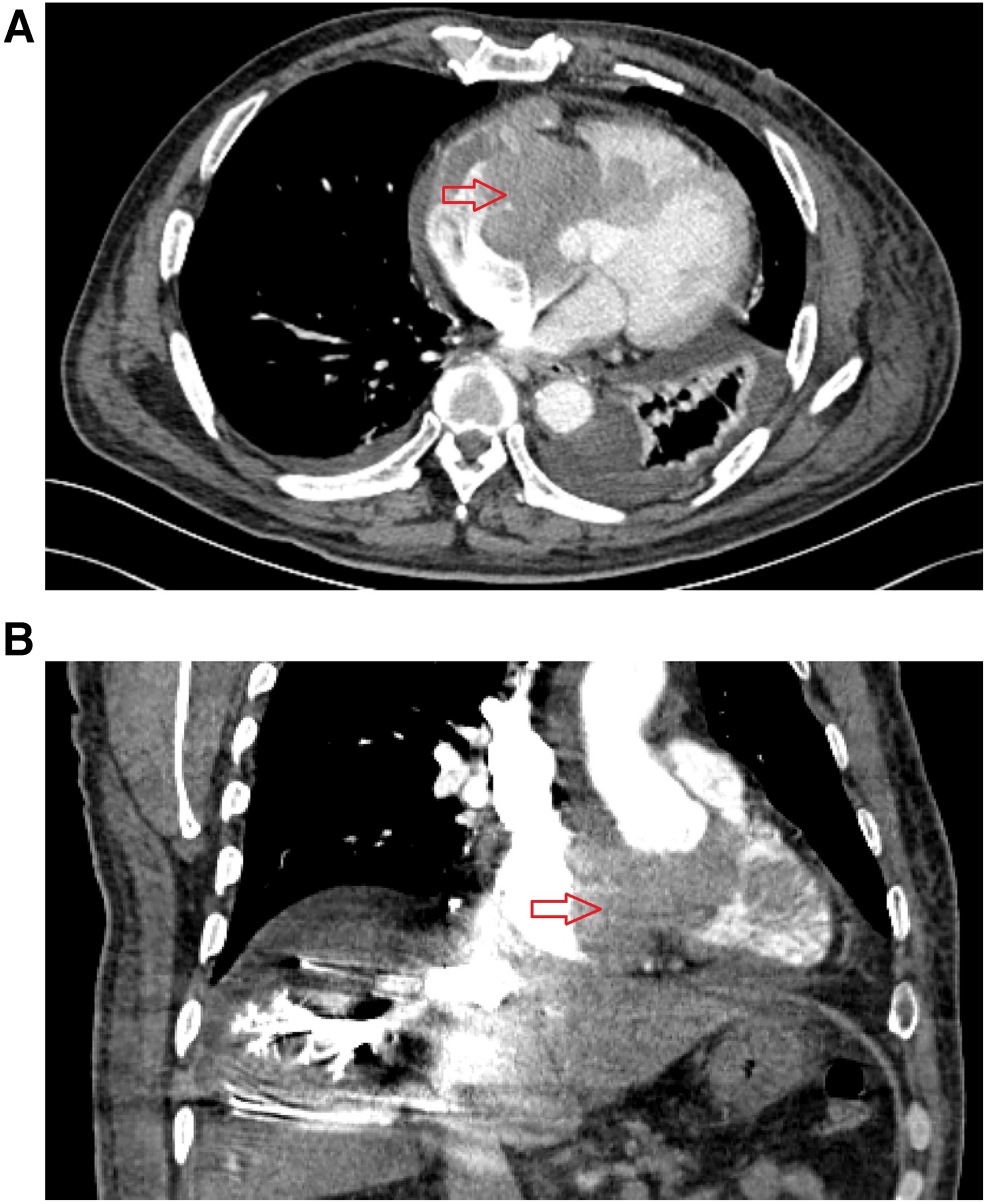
Preoperative enhanced computed tomography results for patient in September 2, 2022. (A) The cross-sectional image shows a mass in the right atrium, with irregular patchy enhancement in the right atrial appendage, right atrium, and right ventricular region, with a CT value of approximately 89 Hounsfield units. The interventricular septum is irregularly thickened. (B) Coronal view shows a mass in the right atrium, uneven opacification in some areas of the superior vena cava, delayed opacification of the inferior vena cava and veins in the right lobe of the liver.
